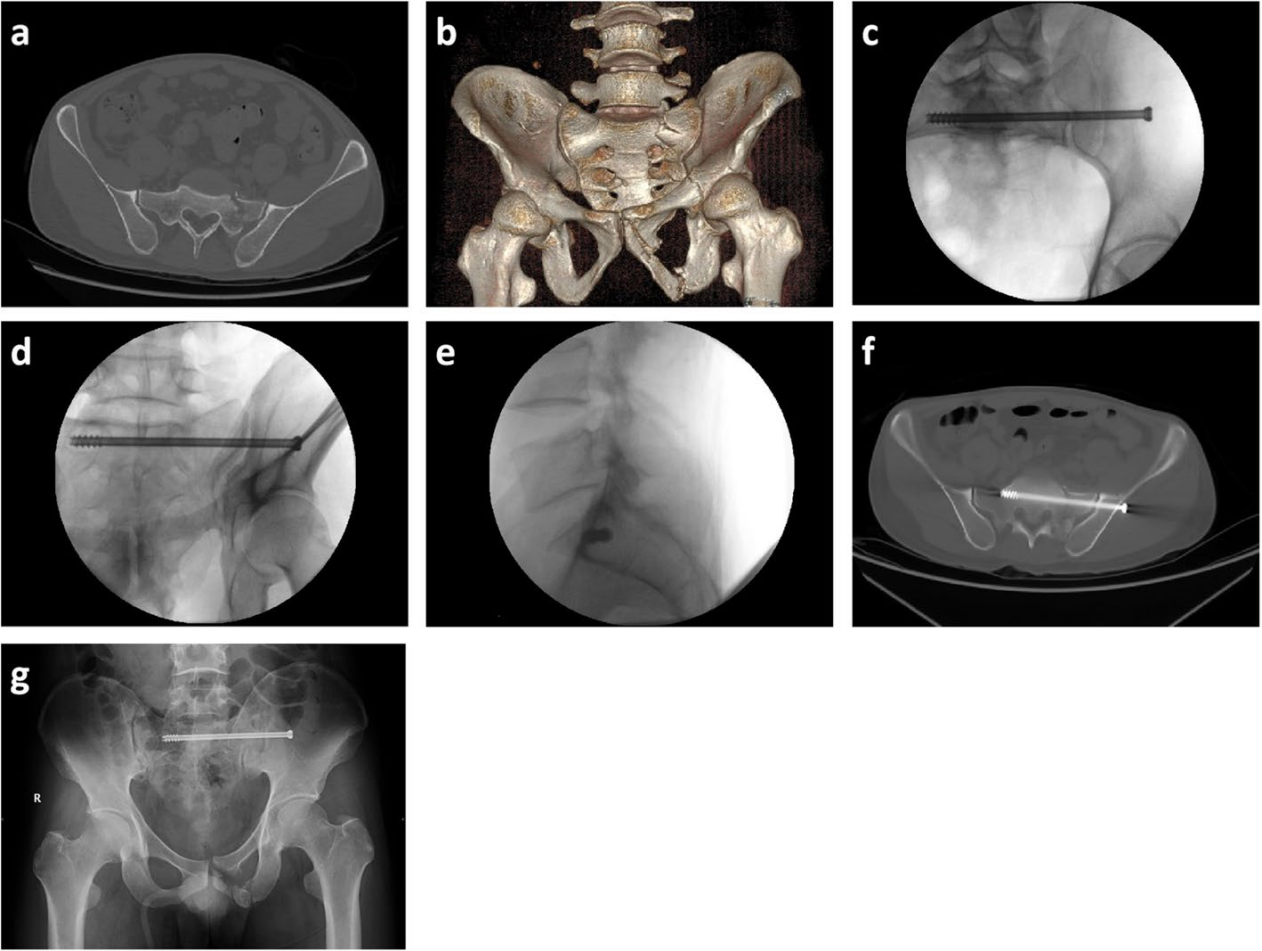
Pre-operative, intra-operative, and post-operative imaging. a-b Pre-operative computed tomography images/ 3D reconstruction for a patient with Tile B2.1 pelvic fractures. c-e Intra-operative imaging (inlet/outlet/lateral view) was used to confirm the IS screw in the target corridor. f-g Post-operative computed tomography axial image and anterior–posterior radiograph computed tomography axial image confirmed the placement of the IS screw
